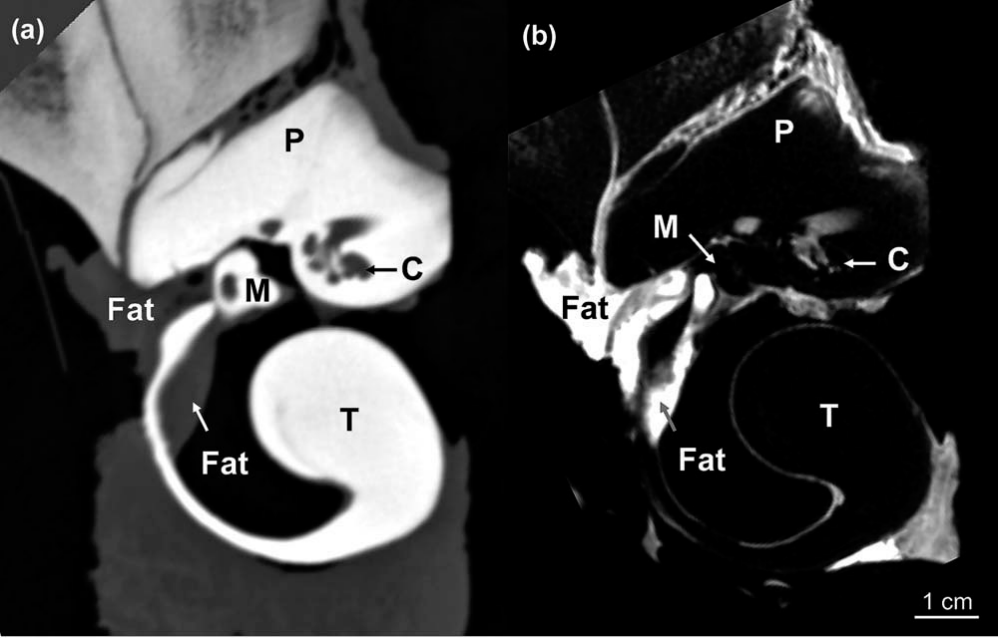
Images of the left tympanoperiotic complex of B-acu17 showing the ear fat inserting into the ears at the lateral aperture between the tympanic and periotic bones (left side of the images) and then attaching to the malleus. The smaller fat body within the tympanic bone is also shown. (a) CT and (b) MRI. Abbreviations: T, tympanic; P, periotic; M, malleus; C, cochlea.
